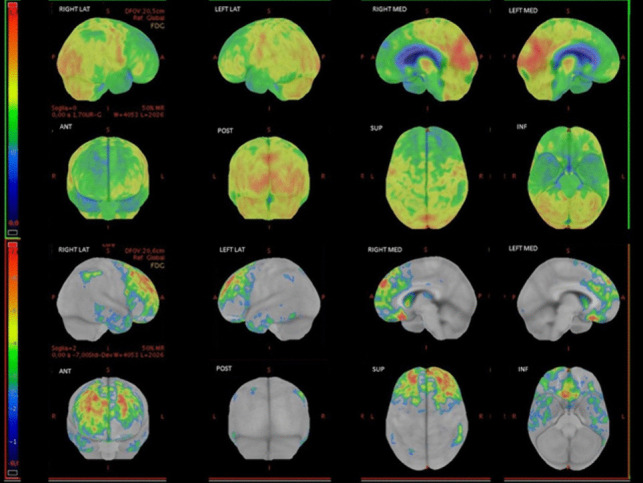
Brain FDG-PET of case II-1, showing predominantly right fronto-temporal hypometabolism. Images have been obtained with CortexID suite (GE Healthcare). Hypometabolic areas appear in cold tones in the upper rows, representing FDG uptake. The lower rows represent the z-score map obtained by comparison with the software database of normal subjects: the higher the z-score (red), the more pronounced the reduction of the metabolism
